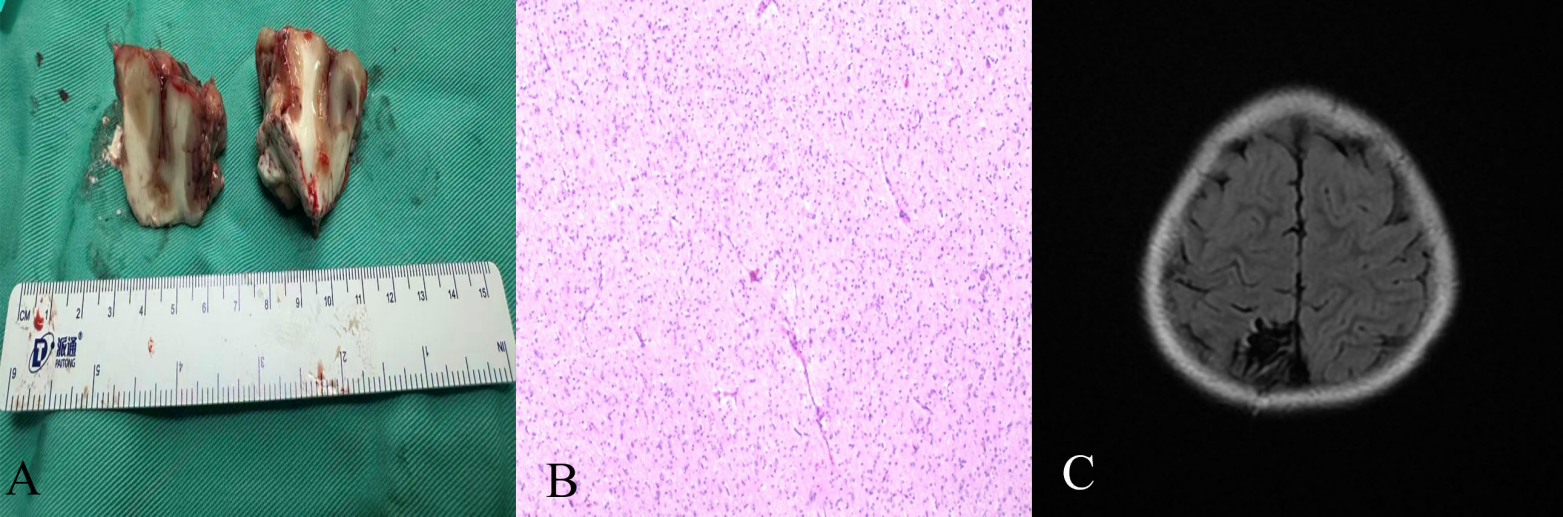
Pathological examination and postoperative imaging of the lesion. (A) Photo of surgical resection of the lesion, showing complete resection of the lesion. (B) Pathology (HE × 100). (C) Postoperative magnetic resonance imaging shows complete resection of the lesion.
